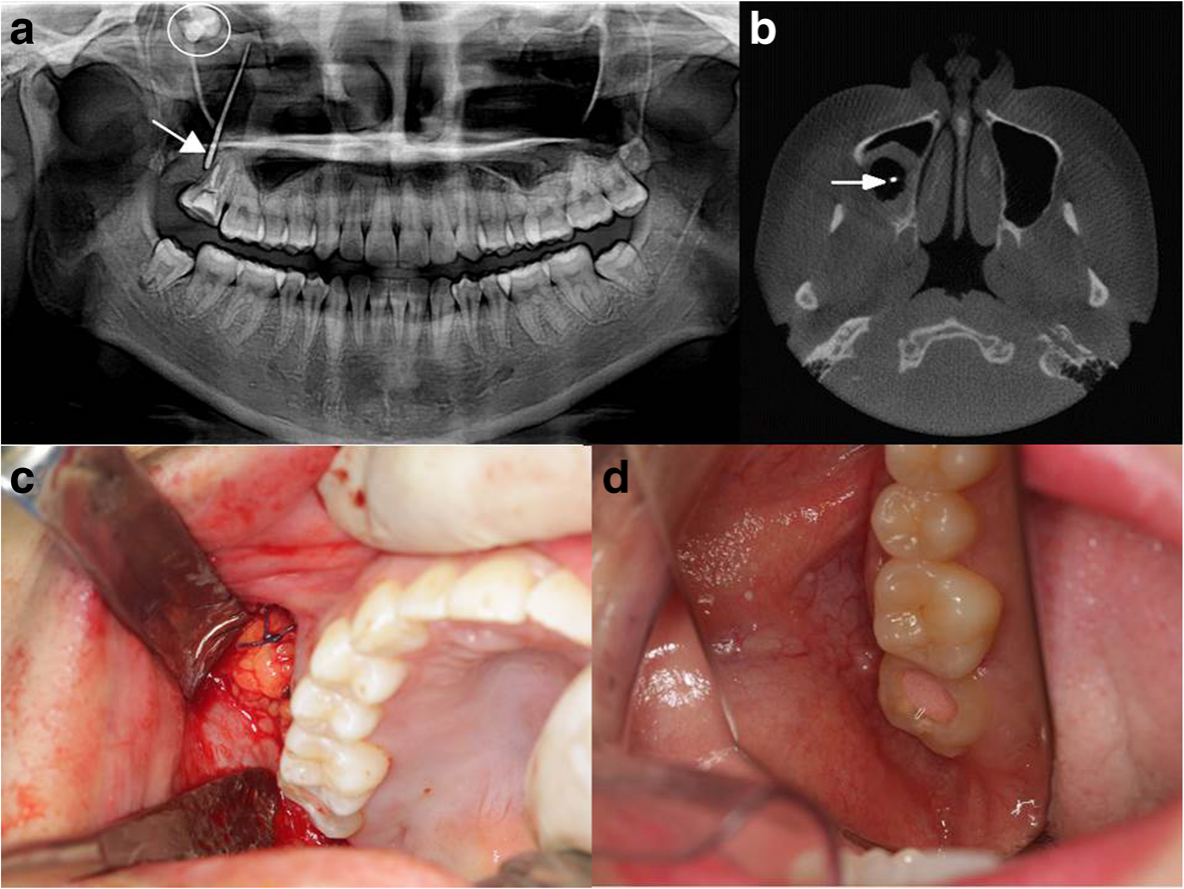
Case 1. a Panoramic view shows an oroantral fistula between the oral cavity and right maxillary sinus cavity (arrow indicates the gutta-percha cone). Additionally, full impaction of no. 18 was observed in the sinus cavity (circle). b Computed tomography showing right sinusitis and gutta-percha cone for examination (arrow). c The defect was reconstructed with pedicled buccal fat pad. d After 3 months, the operation site was successfully closed and well healed
